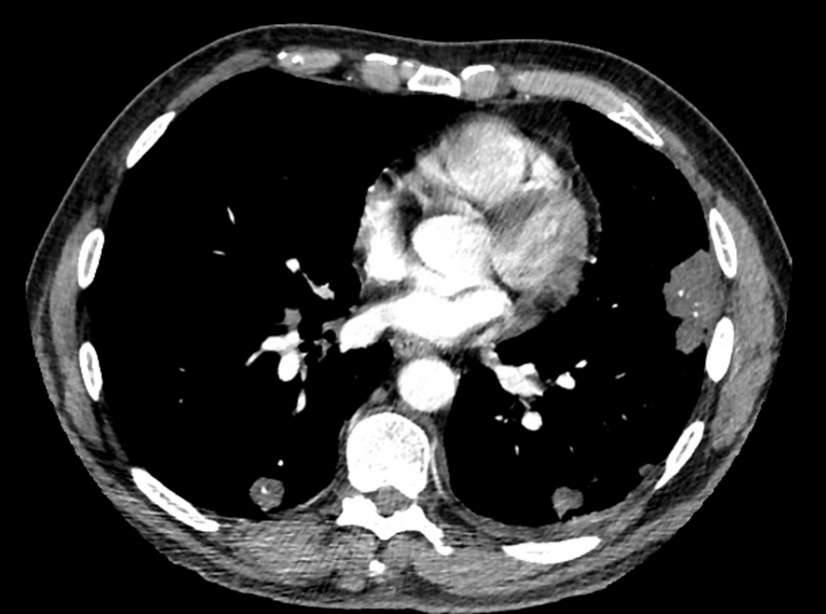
Axial CT image showing partially calcified pleural-based pulmonary nodules.
